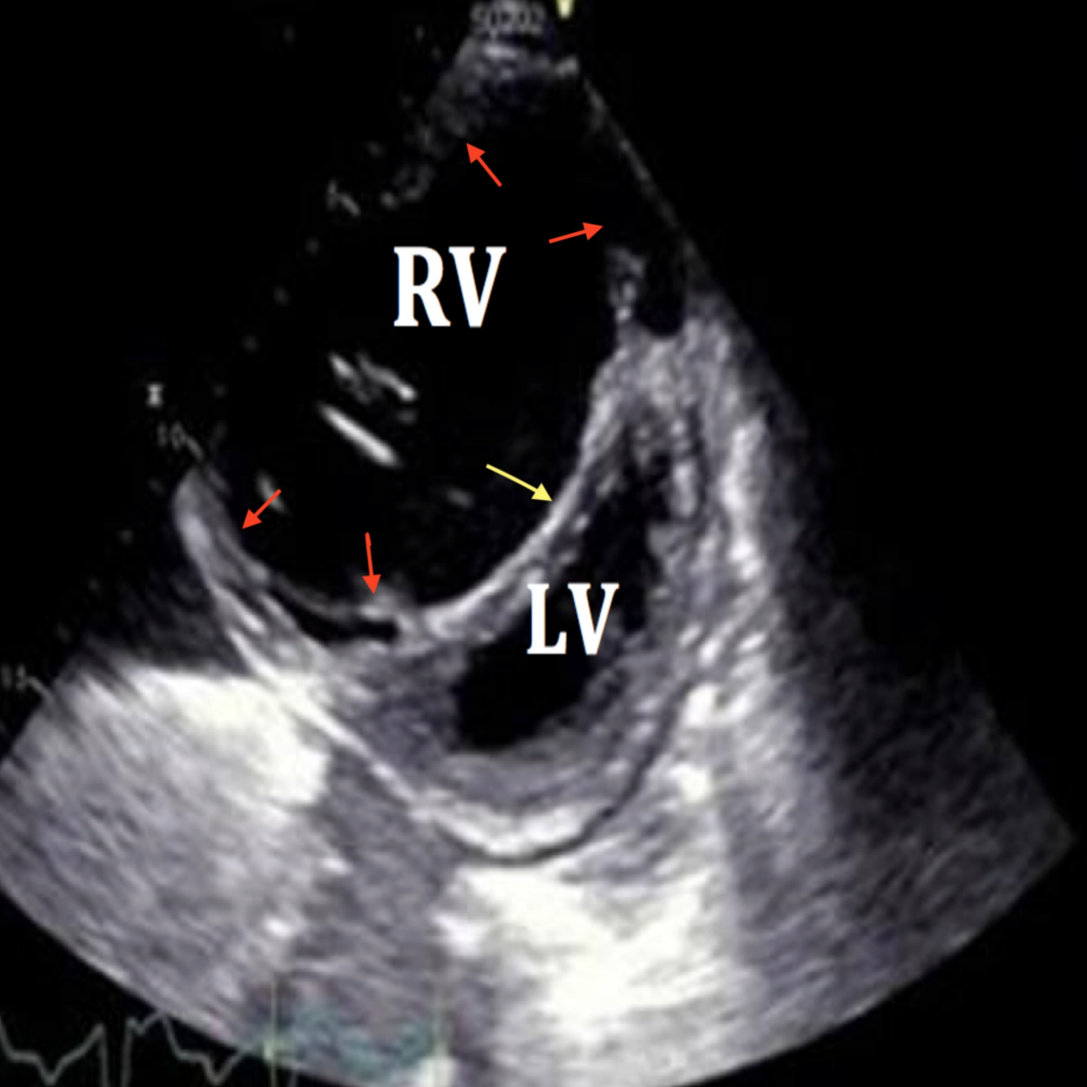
Parasternal short axis view of right ventricular (RV) dilatation (red arrows) and inter-ventricular (LV) septal shift (yellow arrow)
